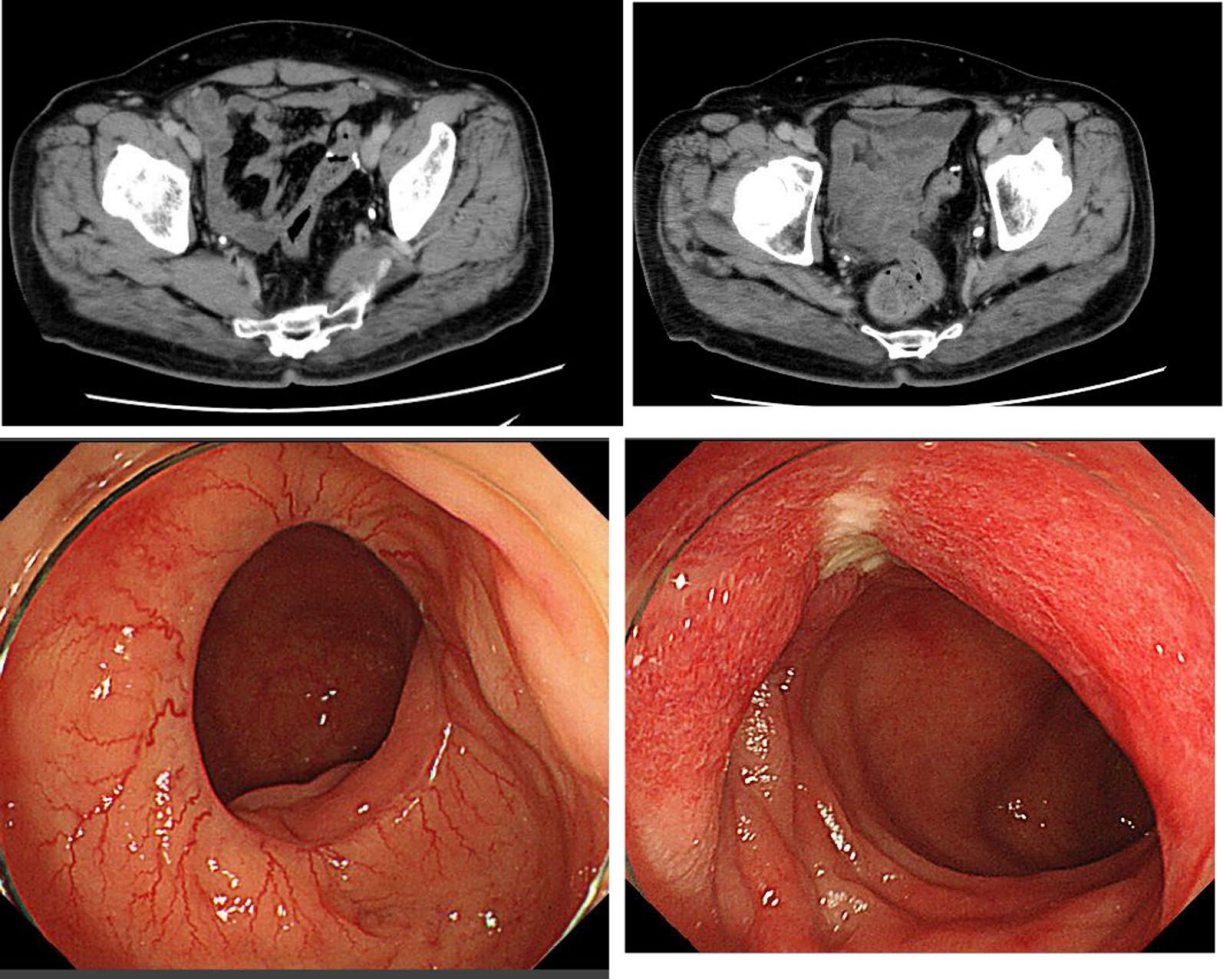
Computed tomography and colonoscopy findings after non-operative management. Computed tomography was performed on the 46th day and colonoscopy on the 35th day. Edematous changes and circumferential ulcers show improvement
